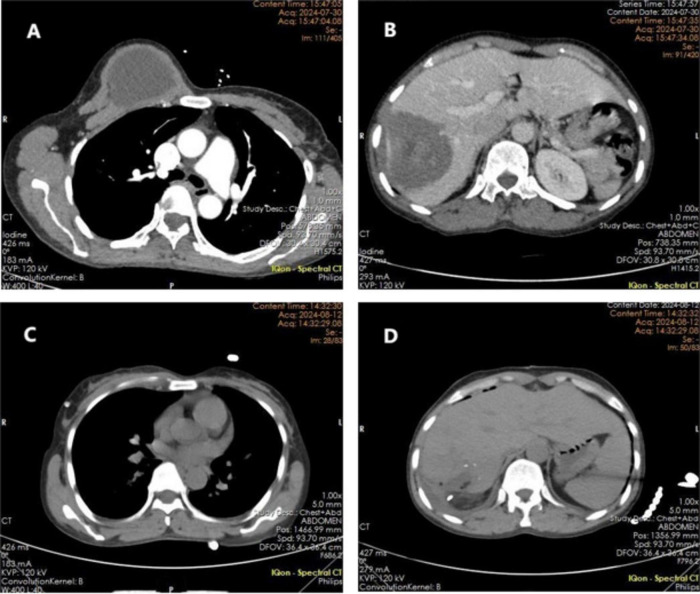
Pre- and post-operative imaging. (A) Preoperative computed tomography (CT) image of the chest wall lesion; (B) preoperative abdominal CT image of the liver lesion; (C) postoperative chest CT image; (D) postoperative CT image of the abdomen.
